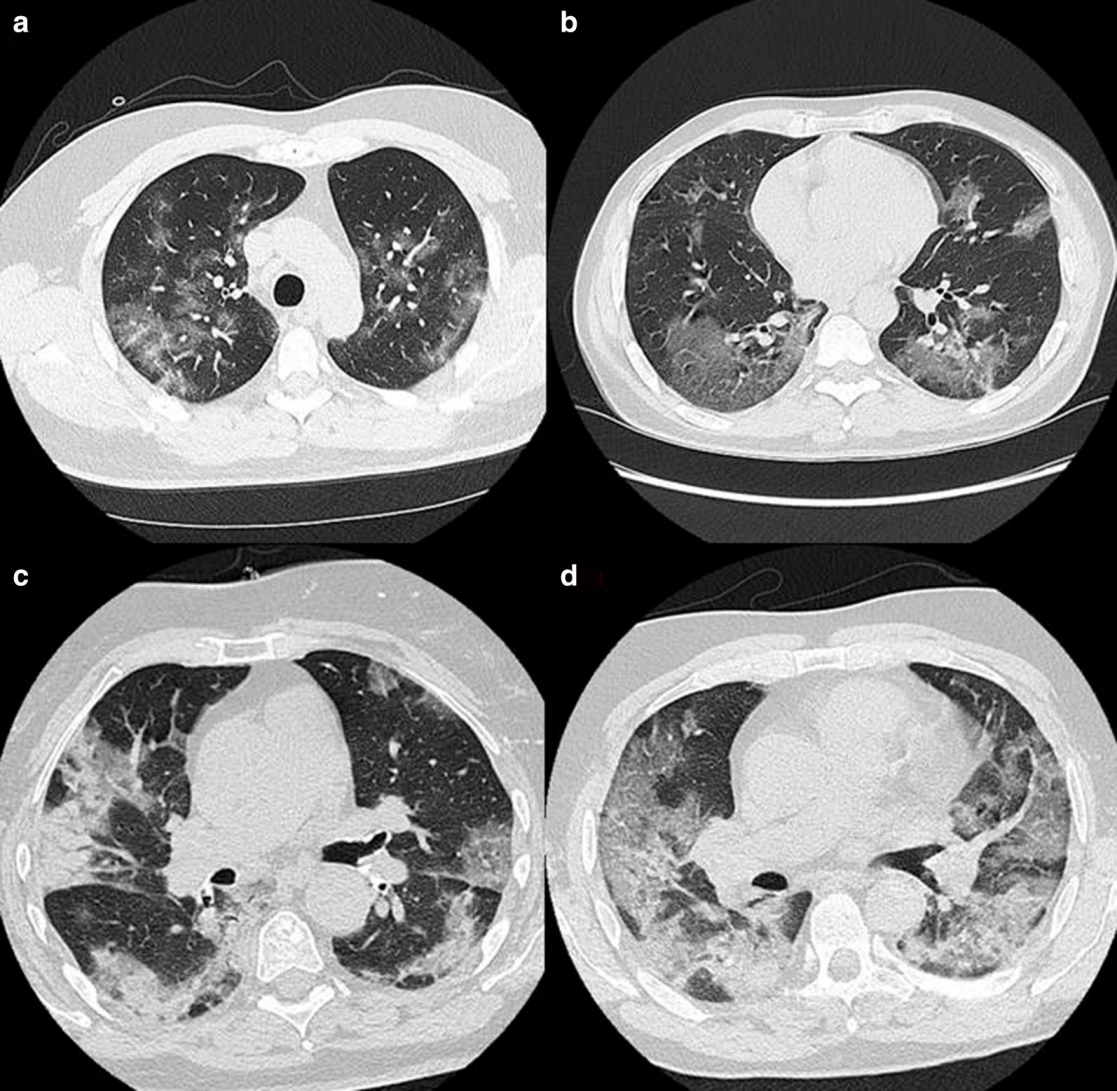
Axial thin-section CT scans in four COVID-19 patients with negative RT-PCR at baseline. a 15-year-old male (6 days after symptom onset to CT scan) shows multiple focal ground-glass opacities associated with linear inter and intralobular septal thickening in upper lobes. b 54 years old male (10 days after symptom onset to CT scan) shows bilateral and predominantly peripheral consolidation pattern in both lower lobes. c 73 years old female (7 days after symptom onset to CT scan) shows extensive bilateral, peripheral, sub-pleural mixed ground-glass opacities in both lower and upper lobes. d 56-year-old male (10 days after symptom onset to CT scan) shows predominantly diffuse consolidation in both lungs
